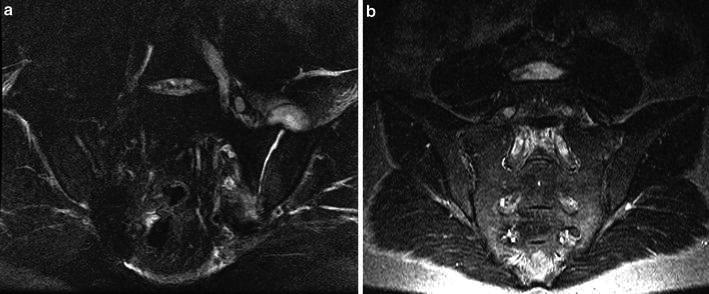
a Case 12: MRI performed 1 week after onset of the patient’s symptoms showed high signal intensity in the left SIJ and iliacus muscle with abscess formation. The patient was operated by combined anterior and posterior debridement. Full mobilisation was allowed after 2 weeks. The patient was satisfied. b FU MRI after 2 months revealed no more abnormal inflammatory signals. At the last FU visit after 80 months, the patient had excellent functional outcome
